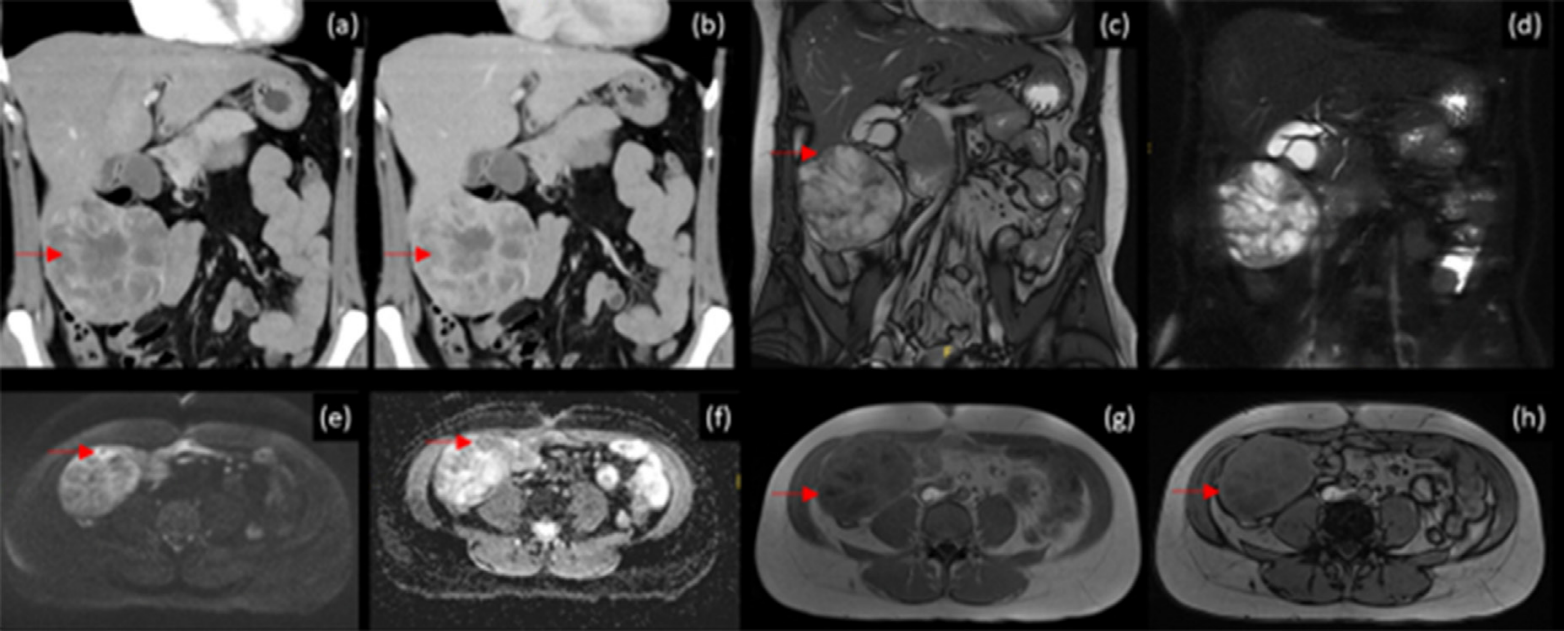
(a) Coronal CT with intravenous contrast in arterial phase demonstrates the exophytic mass at the inferior liver edge with lace-like arterial hyperenhancement. (b) Coronal CT with intravenous contrast in portal venous phase demonstrates progressive nodular enhancement (arrows in (a) and (b)). (c) Coronal *T*
